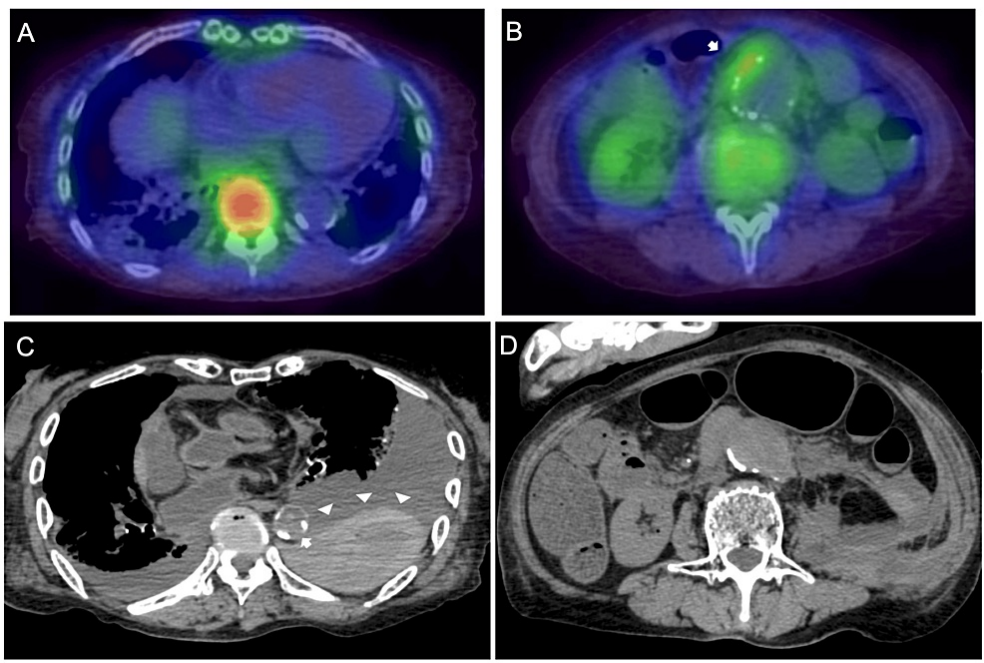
Axial views of gallium scintigraphy of the thorax and abdomen on the ninth hospital day and autopsy CT imaging of the thorax and abdomen. A) Descending thoracic aorta on gallium scintigraphy. No inflammation was detected in the thoracic aorta. B) Infrarenal abdominal aortic aneurysm on gallium scintigraphy. The arrow shows inflammation in the abdominal aortic aneurysm. C) Descending thoracic aorta from the autopsy imaging. The arrow shows that the arterial wall was disrupted, and the arrowheads show a lot of thoracic bleeding. D) Infrarenal abdominal aortic aneurysm from the autopsy imaging. CT, computed tomography.
